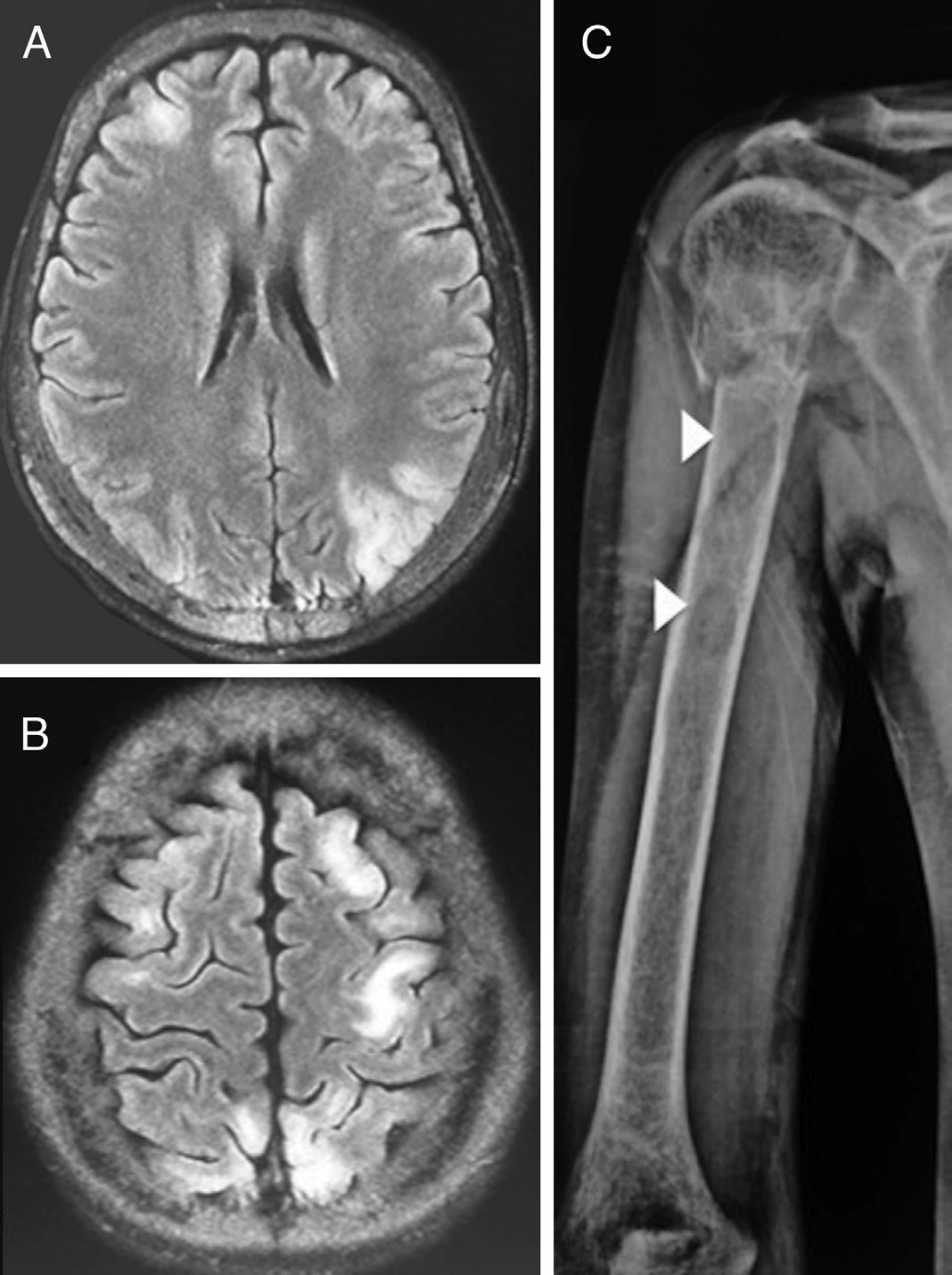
A, B) Magnetic resonance imaging of brain (second case) with T2/fluid-attenuated inversion recovery images showing hyperintensities in the parietal, occipital and frontal regions, more marked on the left side. C) Radiograph of the right shoulder and arm showing a displaced fracture of the surgical neck of the right humerus. Two lytic lesions are seen in the shaft of the right humerus suggestive of brown tumors of hyperparathyroidism (marked in white arrow heads)
